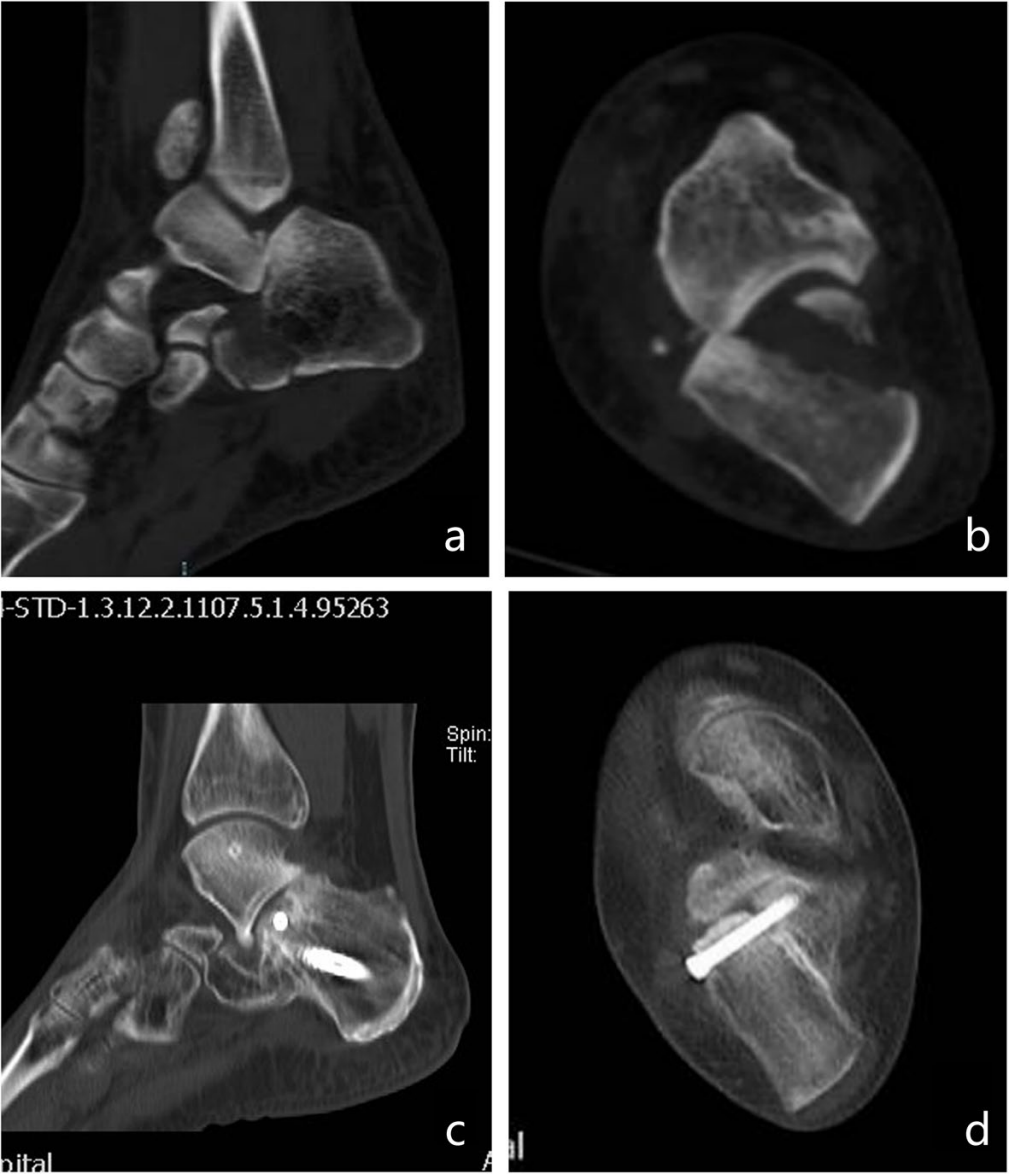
Preoperative CT. sagittal (a), axial (b) views and 1 year postoperative CT.sagittal (c), axial (d) views of a 38 years old female patient who was suffered traumatic arthritis
